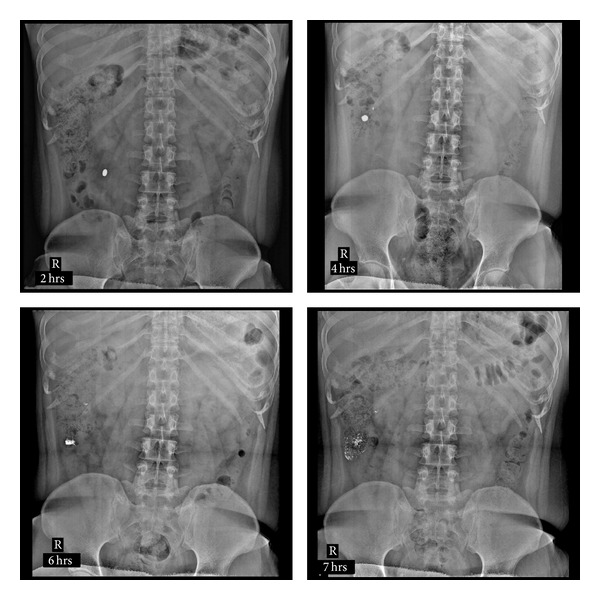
X-ray slides showing compressed coated tablet at different time interval in healthy human volunteer.
